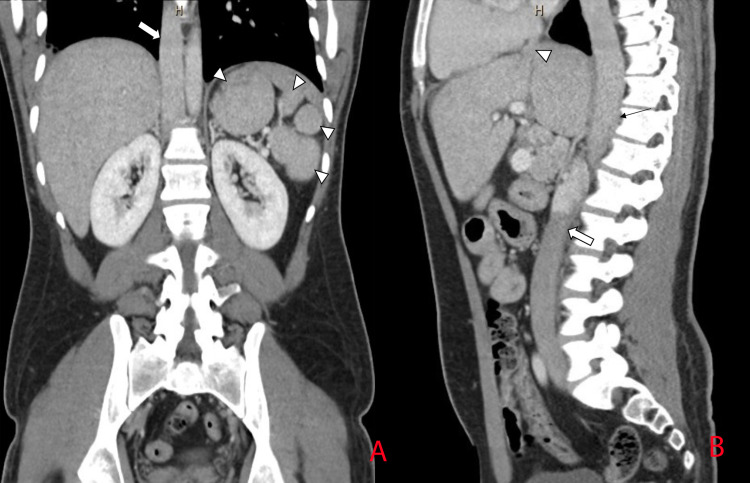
(A) Coronal view of CT scan of the abdomen with IV contrast showing multiple spleens (arrow heads) and the dilated azygous vein (arrow) as a direct continuation of the IVC. (B) Sagittal view of CT scan of the abdomen showing the IVC (wide arrow) draining directly into the dilated azygous vein (thin arrow). Notice the absence of the hepatic segment of the IVC and the hepatic veins draining directly into the right atrium (arrow head). CT: computed tomography, IV: intravenous, IVC: inferior vena cava.
